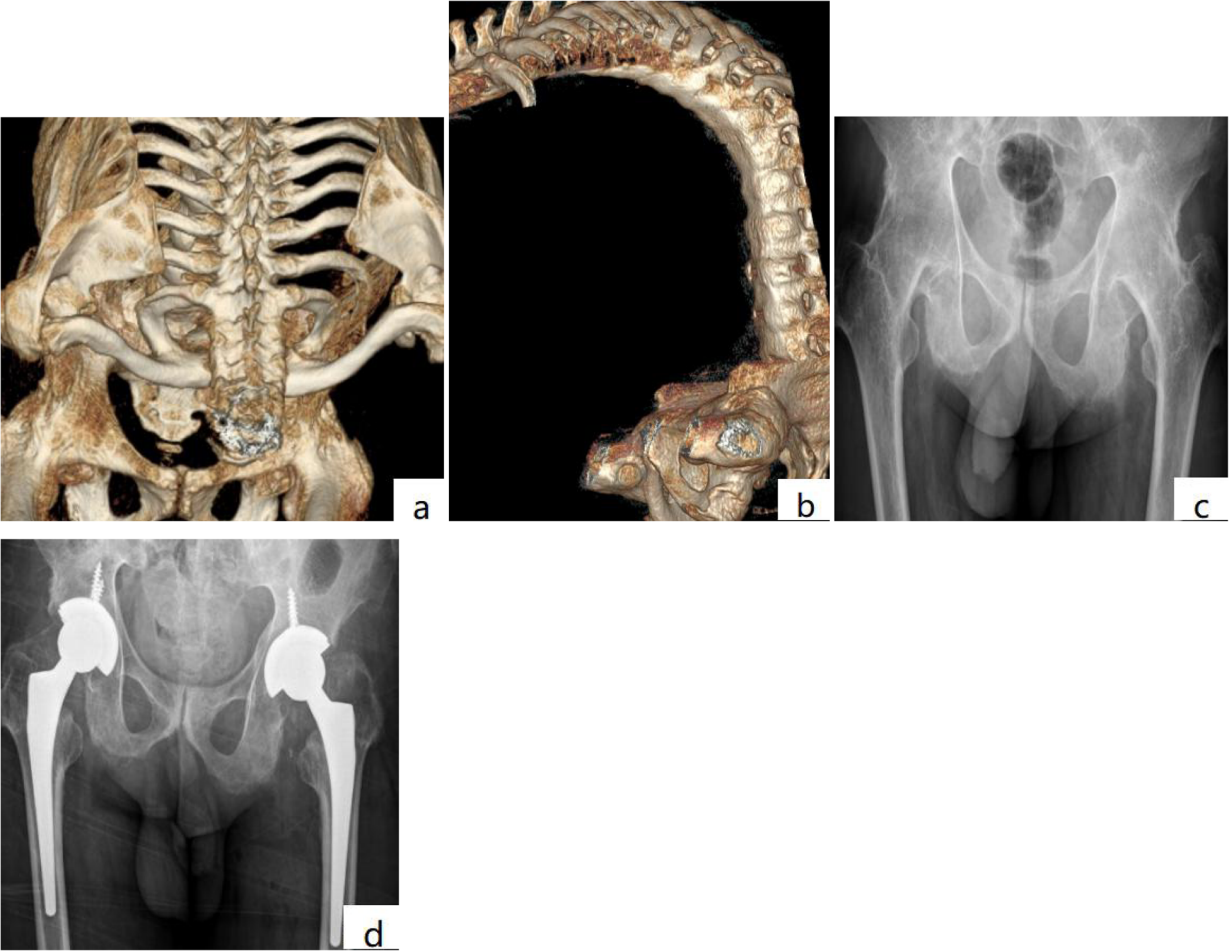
a, b Three-dimensional reconstruction showing AS with hip flexion ankylosis and kyphosis deformity. c The radiographs showing bilateral bony ankylosis with 0° range of motion. d Postoperative radiograph of the patient 5 years after cementless THA
